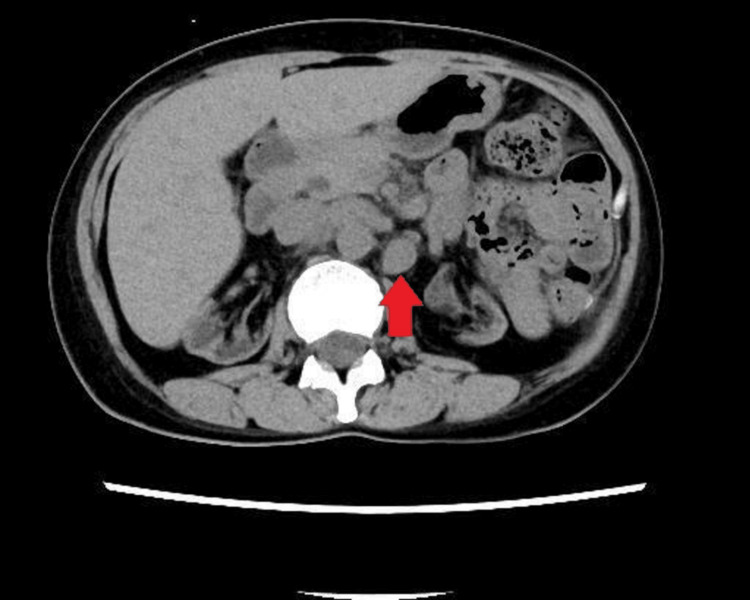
Contrast-Enhanced CT Before Rituximab Therapy Contrast-enhanced computed tomography performed at the time of referral to our institution before initiation of rituximab therapy demonstrates intra-abdominal lymphadenopathy (arrow), suggestive of lymphoproliferative disease.
